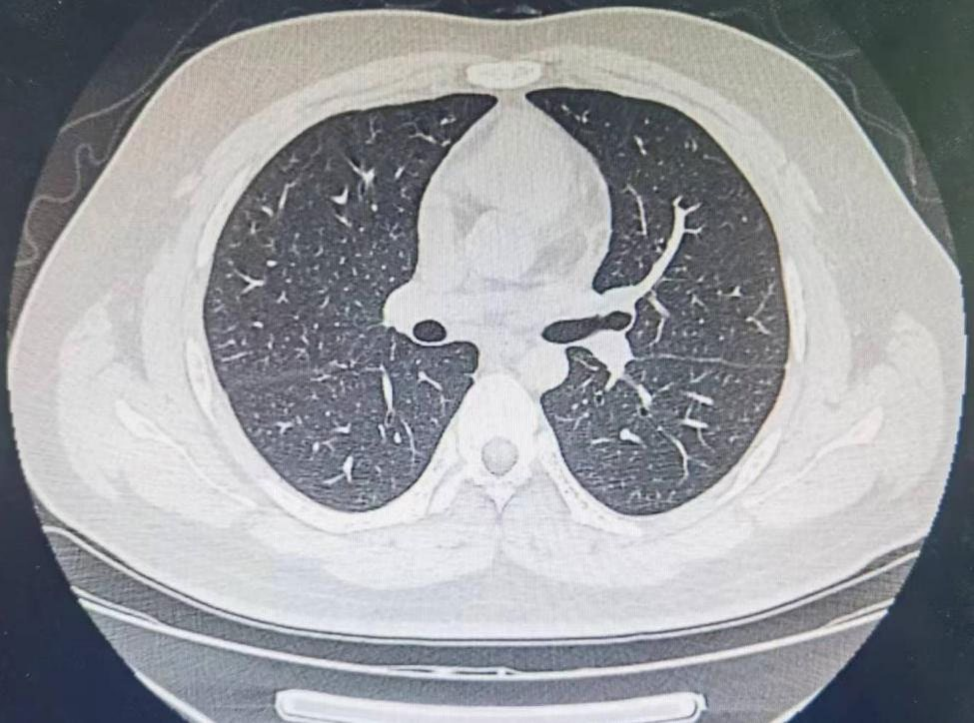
Postoperative chest CT showed patency of the left main bronchus
